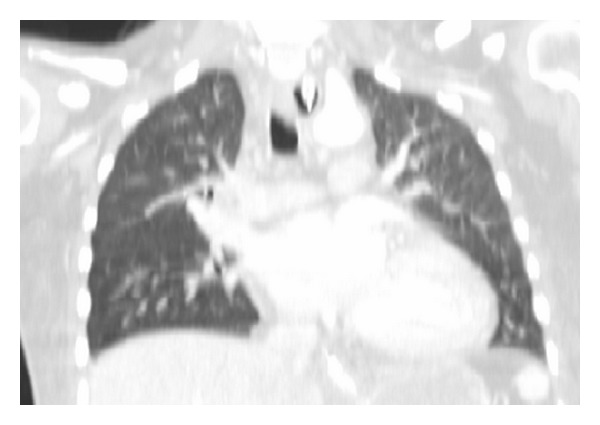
CT thorax four months after treatment demonstrates improvement in airspace opacities.
